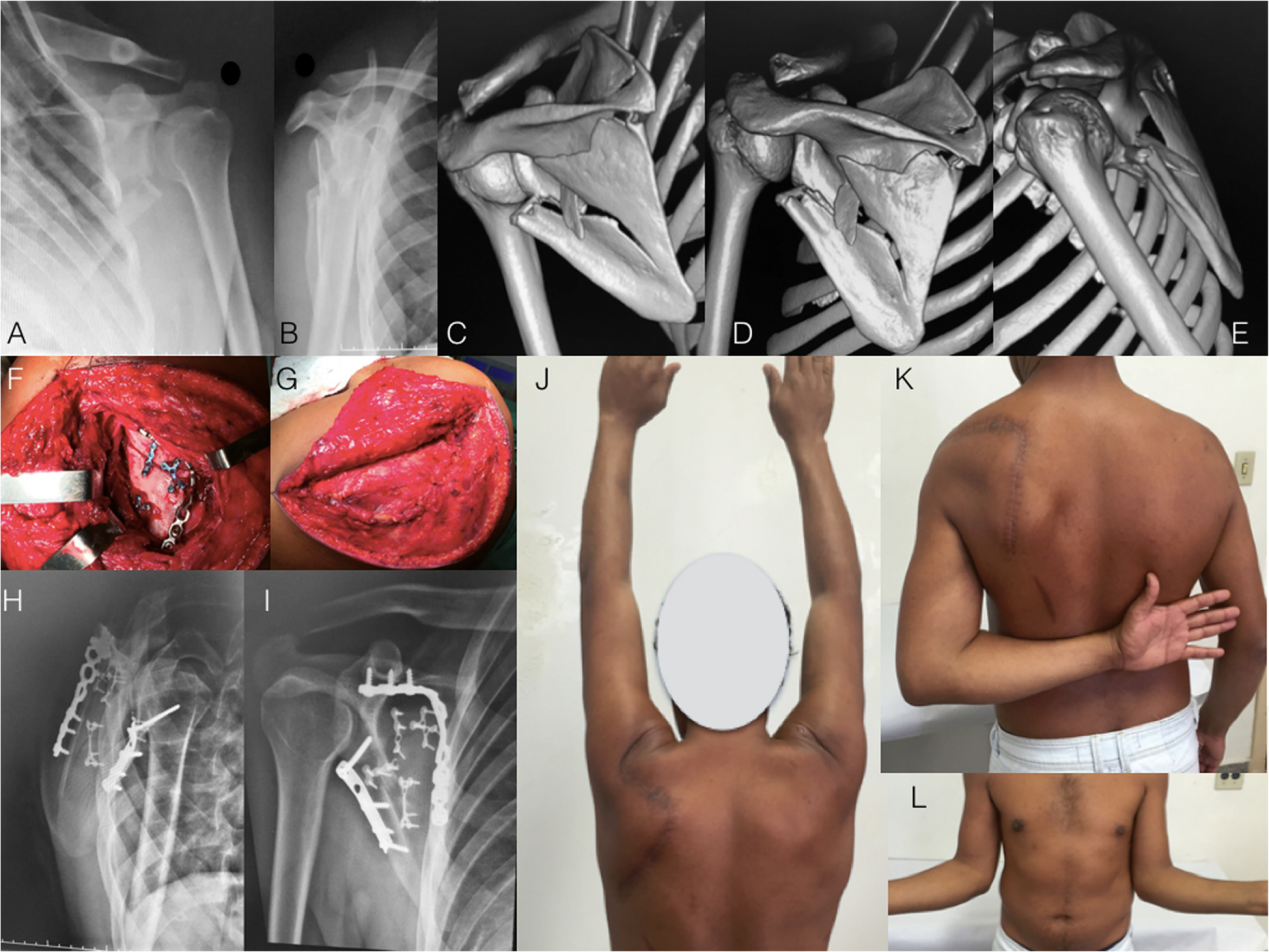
a and b: Radiographs of the left shoulder of a 34-year-old male patient who suffered a motorcycle accident and presented a severely displaced and comminuted infraspinous fracture of the scapula body. c, d, and e: Computed tomography with three-dimensional reconstruction. Observe the angulation of the scapula body and the degree of glenoid medialization. f: Perioperative photography depicting the classic Judet approach. Observe the extensile detachment of the infraspinatus muscle. g: Perioperative photography showing the closure and infraspinatus muscle. h and i: Radiographs after three months showing the fracture healing after fixation with one-third tubular plate at the lateral pillar and a twisted reconstruction plate at the medial pillar of the scapula. Fragment-specific fixation using 2.0-mm minifragment plates was also performed. j, k, and l: Postoperative photographs showing complete range of motion recovery after three months of surgery
